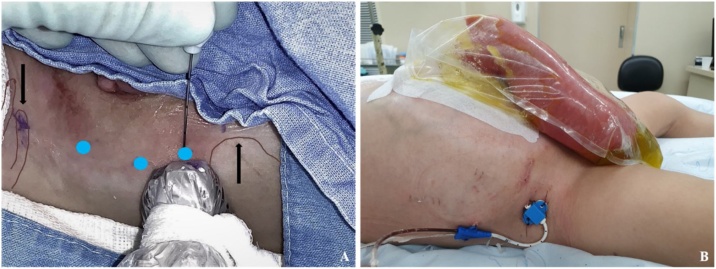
A: BTA application sites ultrasound guided (blue dots) between and 12° rib and anterosuperior iliac spine (black arrows). B: PPP site of catheter insertion.
